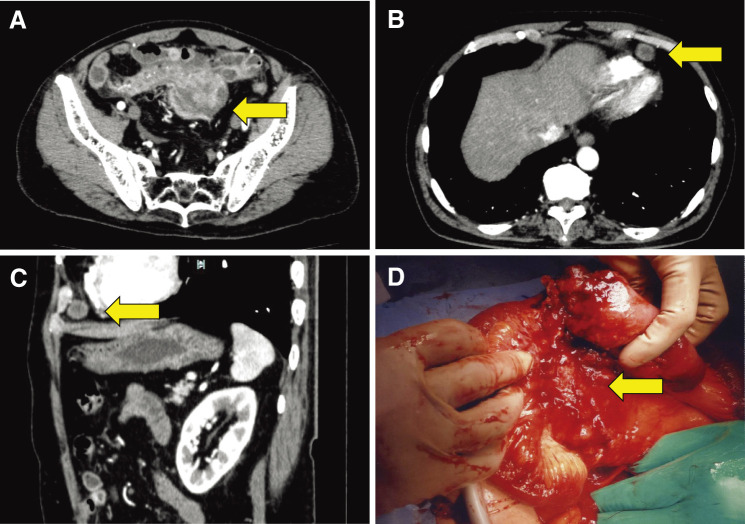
Contrast-enhanced CT (CECT) images upon admission (Day 1) and intraoperative photograph. CECT revealed a solid tumor measuring 6 cm in maximum diameter with moderate vascularity located in the mesentery of the terminal ileum (A, arrow) and a mediastinal tumor measuring 1.5 cm in diameter (B and C, arrow). A soft, bulky tumor situated in the mesentery involved the terminal ileum (D, arrow).
